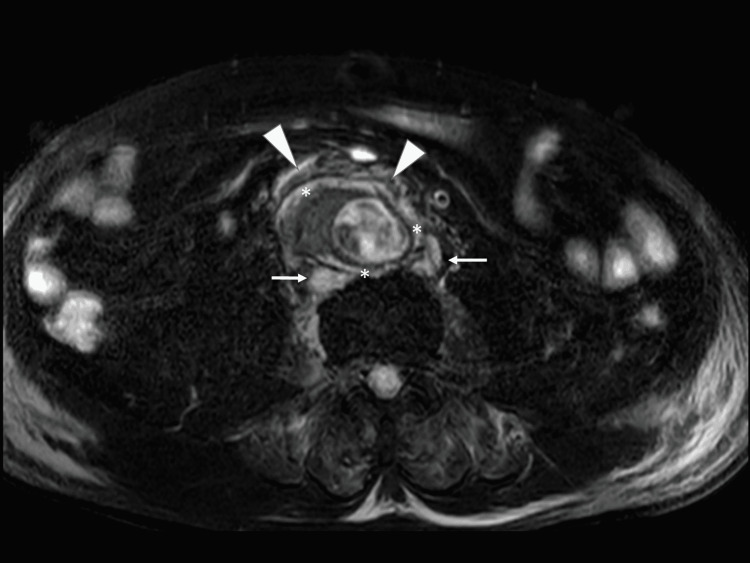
Abdominal short TI inversion-recovery MRI on day five The MRI showed a high abdominal aortic aneurysm with an inflammatory aortic wall (*), swelling of the paraaortic lymph nodes (arrow), and periaortic fat inflammation (triangle).
